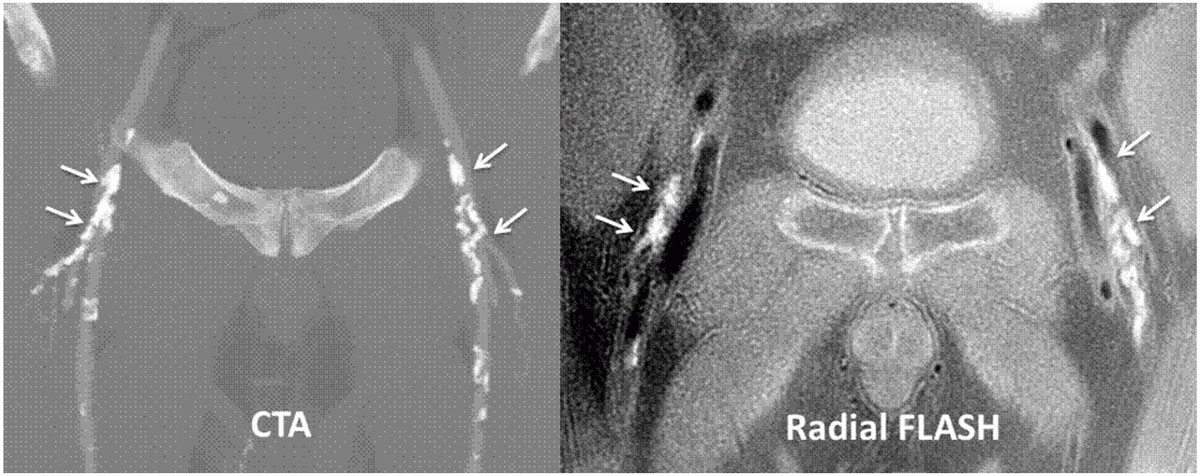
Comparison of maximum intensity projection images from CT angiography (left) with minimum intensity projection images (right, displayed with inverted gray scale) from radial FLASH. There is excellent correspondence in the appearance of the ilio-femoral vascular calcifications between the two imaging modalities.
